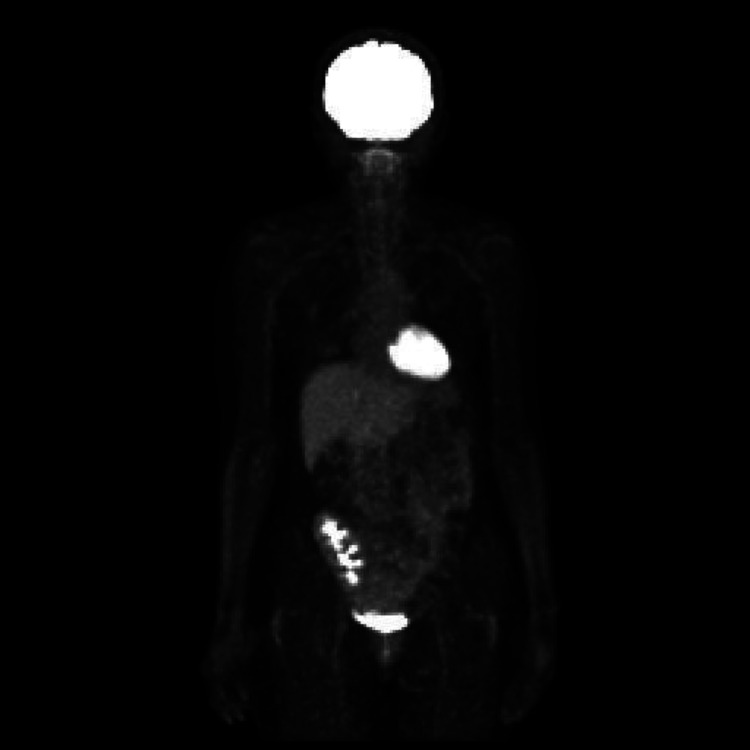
FDG-PET/CT After R-CHOP Therapy Follow-up fluorodeoxyglucose positron emission tomography/computed tomography (FDG-PET/CT) after completion of rituximab + cyclophosphamide, doxorubicin, vincristine, and prednisone (R-CHOP) chemotherapy demonstrates complete metabolic remission with no abnormal FDG uptake.
